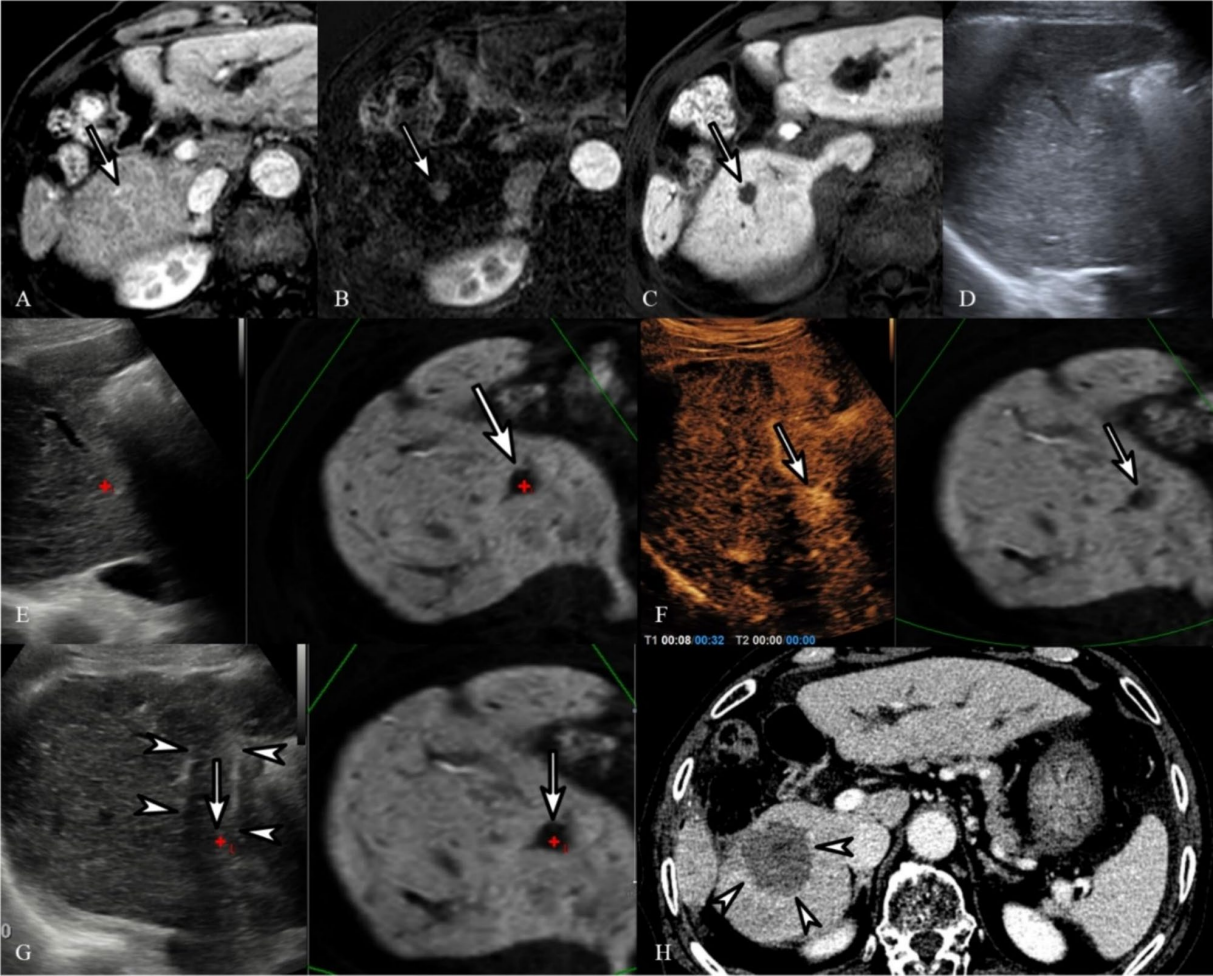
Images of using SonoVue in radiofrequency ablation. Images of a 76-year-old man who had a 1.5-cm hepatocellular carcinoma (HCC) and hepatitis B virus-related liver cirrhosis and underwent CEUS-CT/MRI FI-guided radiofrequency ablation (RFA) with the use of SonoVue as a contrast agent. Arterial phase (A) and its subtraction MRI (B) demonstrates a small arterial-enhancing HCC (arrow) in liver segment 6. (C) In the hepatobiliary phase (20-min delayed phase), the tumor at segment 6 of the liver showed a clear defect (arrow). On B-mode US (D) and following fusion imaging (E), it was not possible to identify the HCC on B-mode US at the corresponding site with fused MRI (arrow). Therefore, the index tumor was assigned a conspicuity score of 1, since it was definitely unidentifiable on fusion imaging. (F) Arterial-phase imaging obtained post-SonoVue injection showed a small enhanced lesion (arrow) that could be clearly identified at the corresponding location on fused MRI. (G) Radiofrequency electrodes (arrowheads) were inserted into the index tumor (arrow) with CEUS-added fusion imaging guidance. (H) Portal-phase CT obtained immediately post-RFA demonstrates technical success and sufficient ablation margins (arrowheads). LTP was not identified at the site of ablation after 10 months of the procedure
